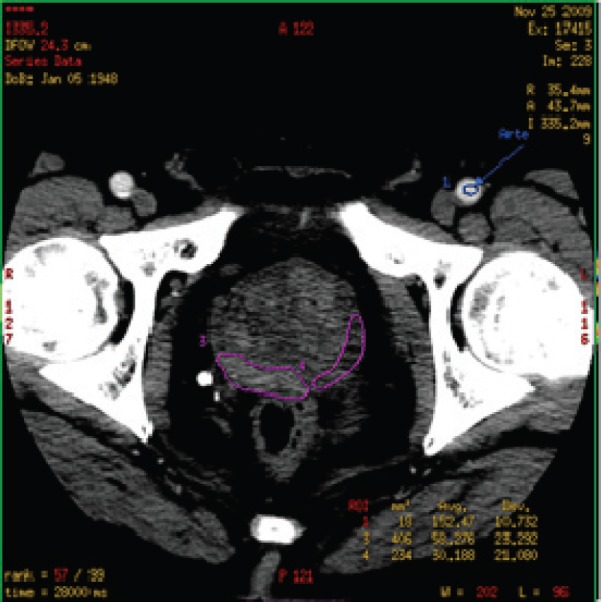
Region of interest (ROI) in prostate CT perfusion: the manually drawn ROI containing malignant tissue (purple contour on the right side of prostate gland) and healthy tissue (purple contour on the left side of prostate gland).
